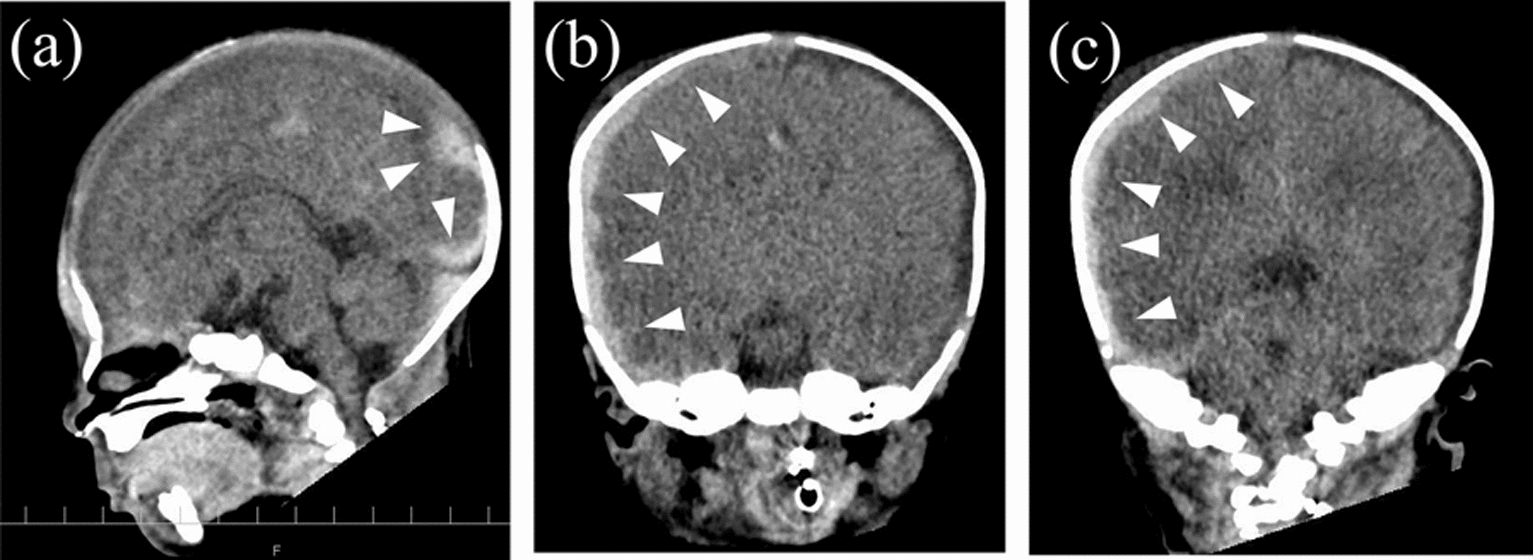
Cranial computed tomography at 14 hours after birth: a sagittal view, b coronal view (anterior horn of lateral ventricles), and c coronal view (posterior horn of lateral ventricles). Extensive hyperdense lesions indicative of hemorrhage present on the right side (arrowheads)
